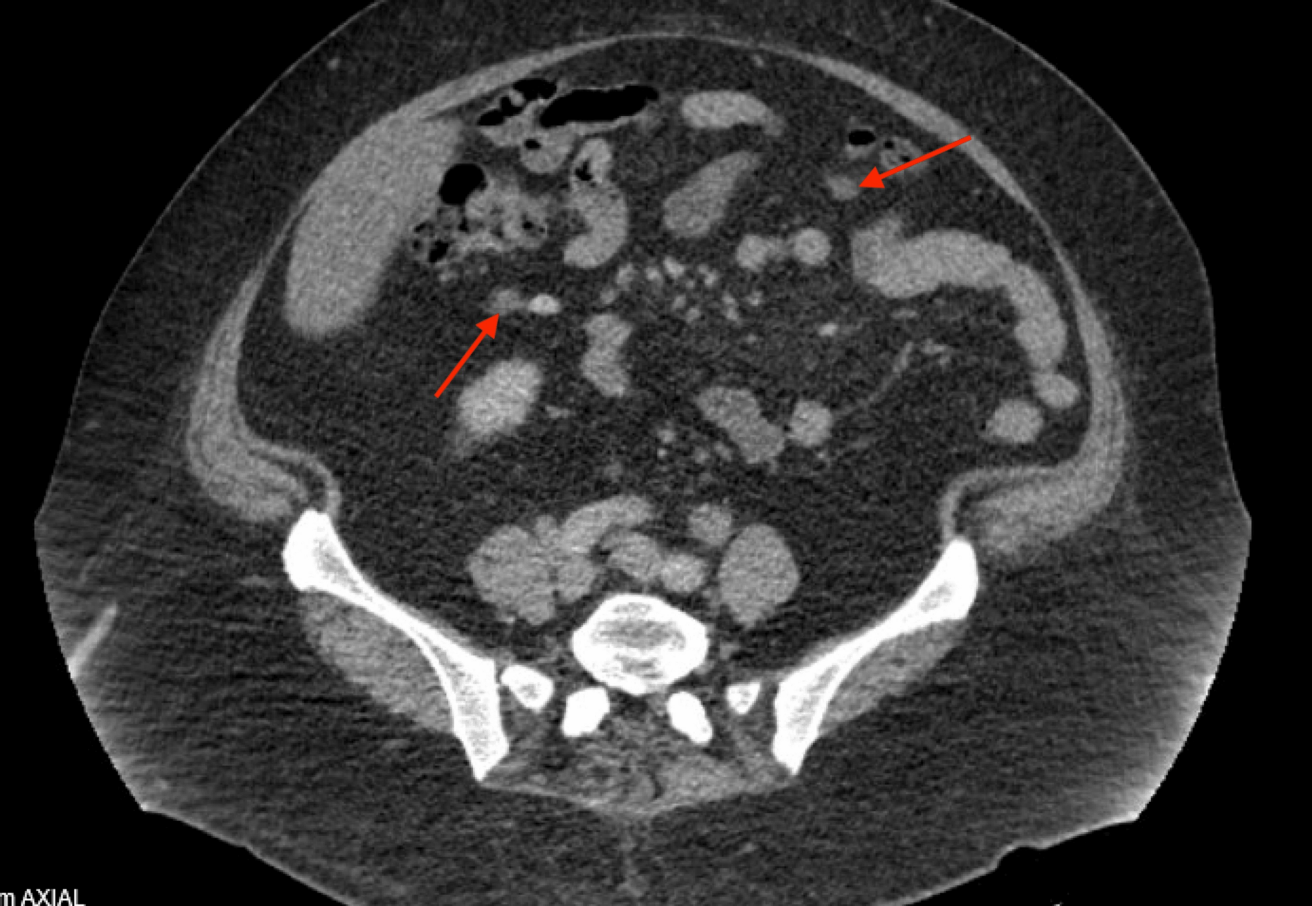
Initial CT scan of the patient's abdomen and pelvis showing evidence of mesenteric and retroperitoneal lymphadenopathy. CT, computed tomography
